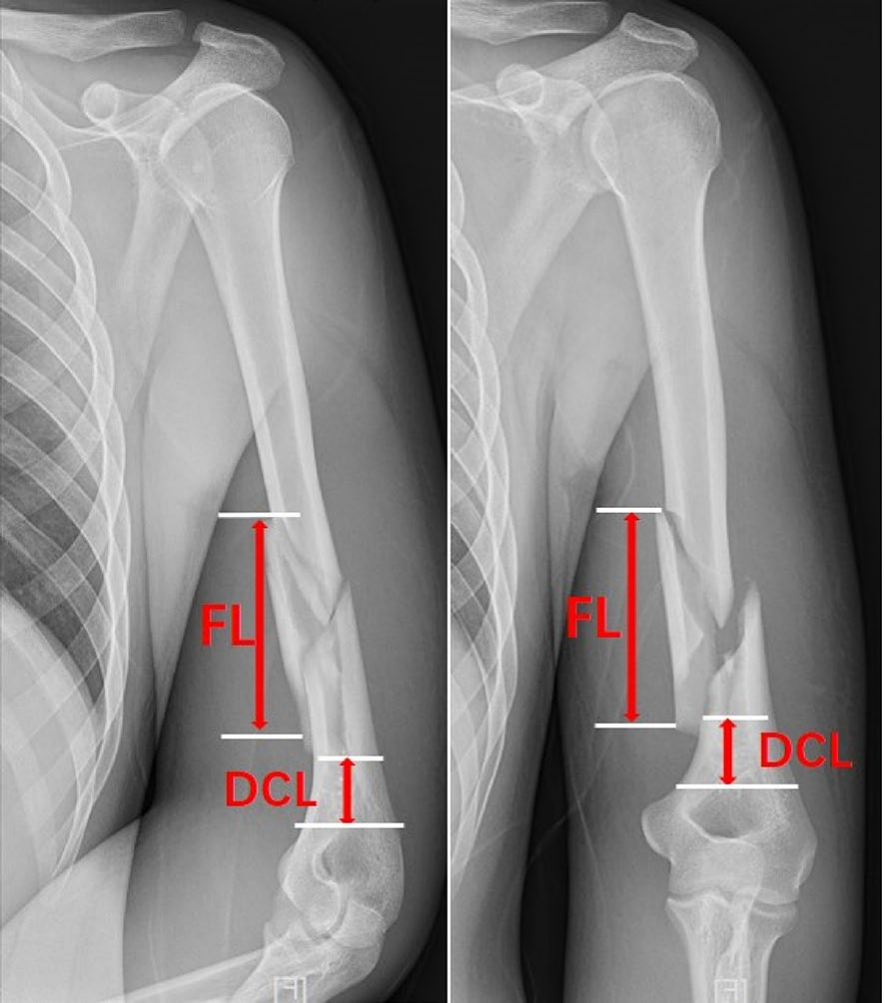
Positive and lateral X-ray of a typical distal third diaphyseal fracture of humerus: The mean fracture length (FL) is defined as the longest fracture line observed in the X-ray image; The distal cortical length (DCL) is defined as the distance between the upper edge of the olecranon and the distal end of the fracture line.
